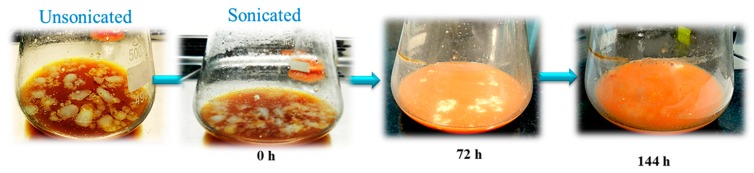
Appearance of fat from ultrasonicated clarified butter sediment waste medium (CBM) during utilization by *R. kratochvilovae* over a cultivation period of 144 h.
